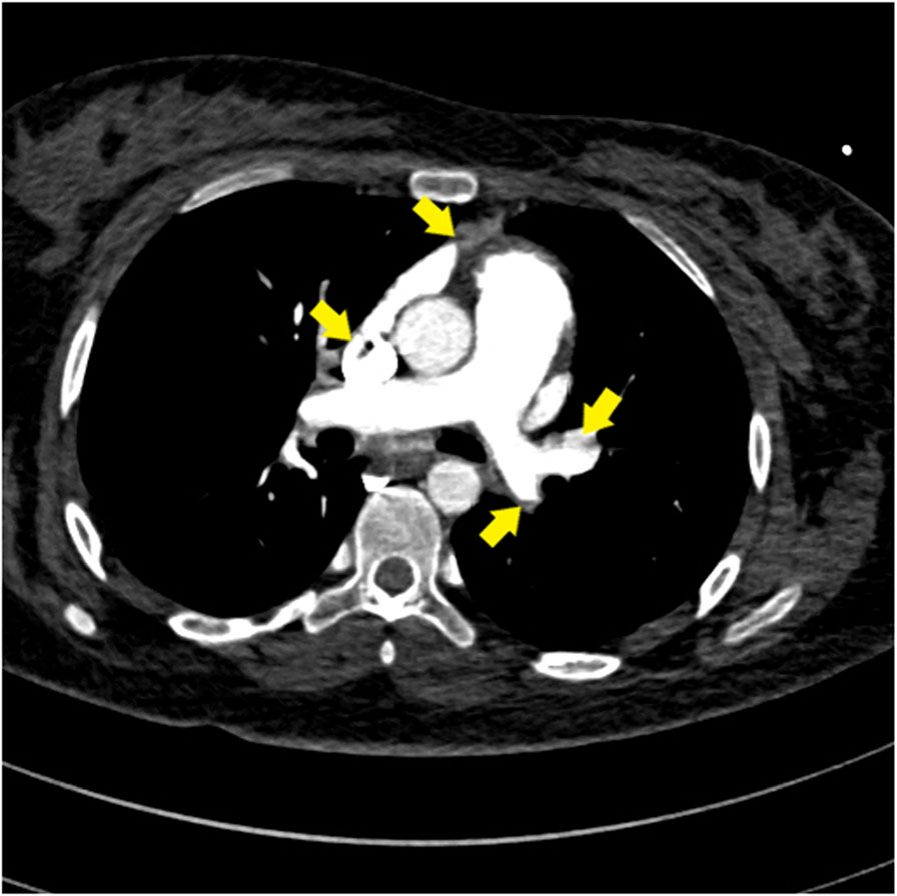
Multi-slice CT pulmonary angiography showing normal major pulmonary artery branches with occluded some peripheral pulmonary branches
